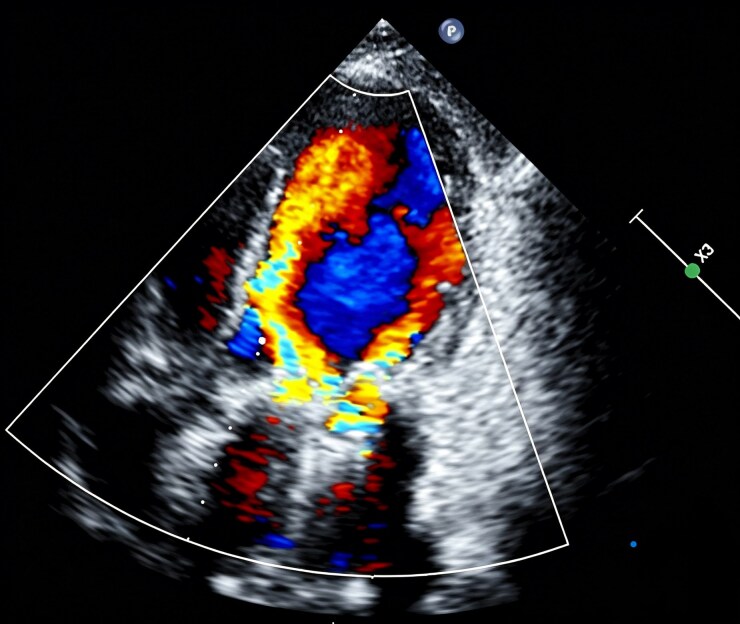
Ultrasound image of mechanical valve stenosis.
